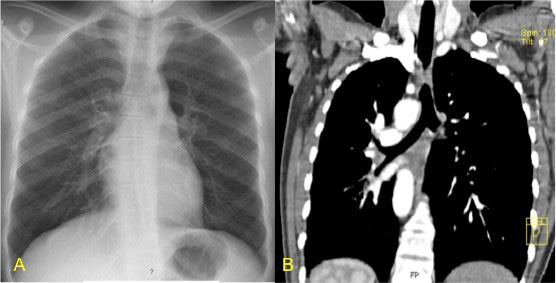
A. The postero-anterior plain chest radiograph, and B, the coronal
